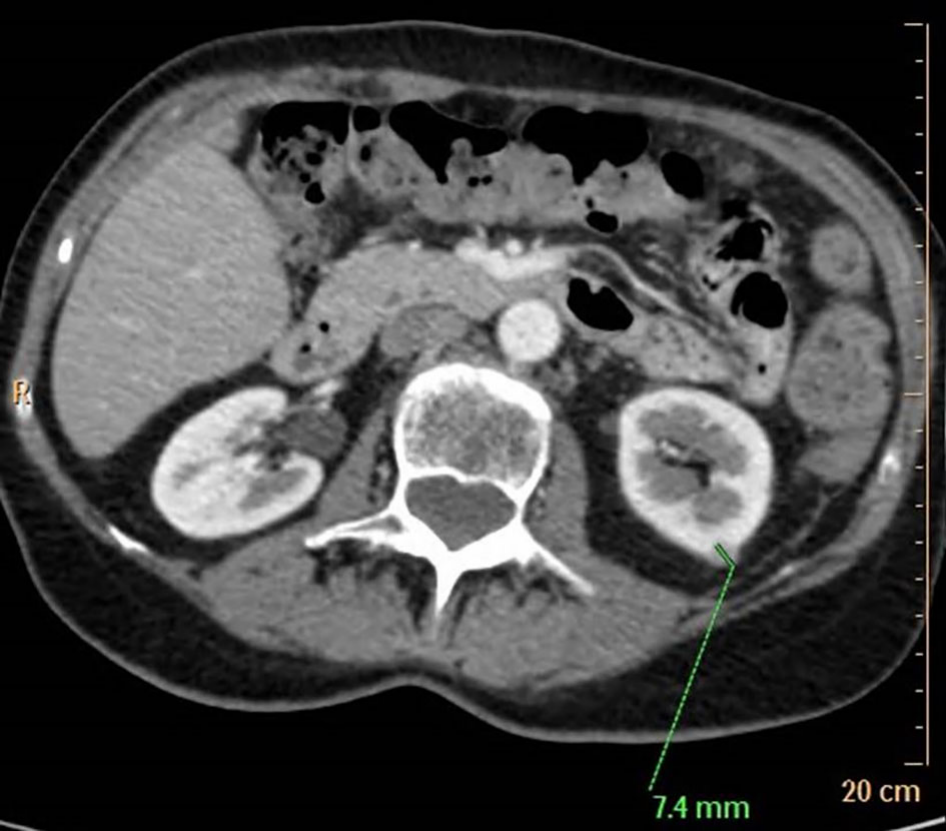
7.4 mm metastasis in the left renal cortex. Contrast-enhanced computed tomographic scan.
